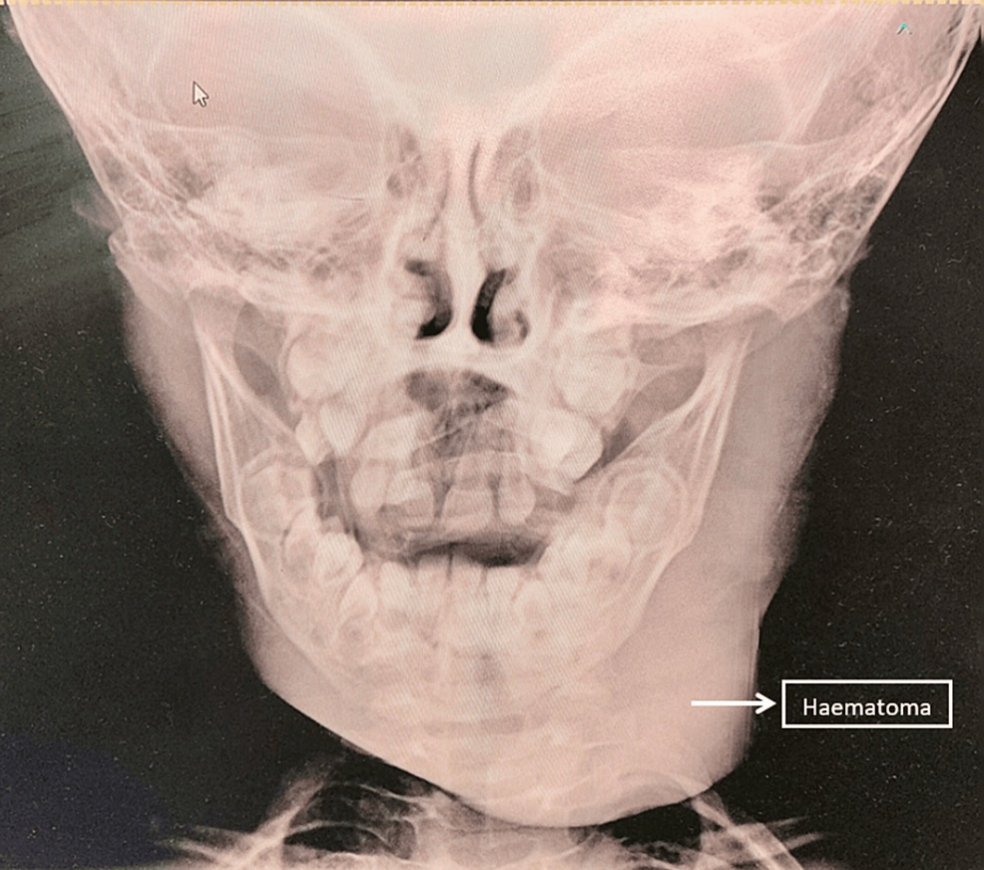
Haematoma seen on the anterior-posterior view of the neck and face X-ray
